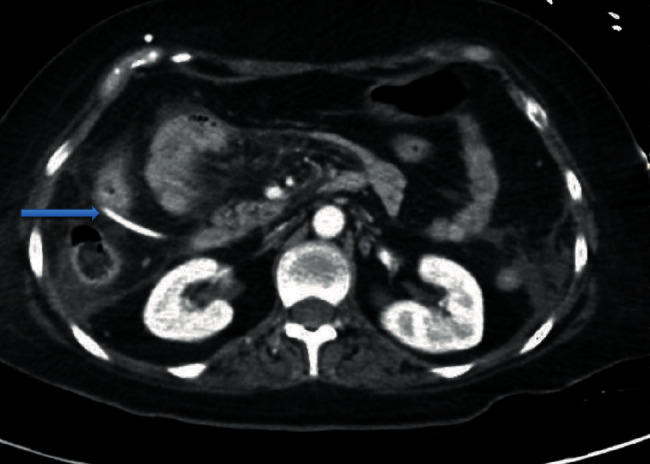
CT of the abdomen showing dilated loops of bowel concerning for enteritis and ventriculoperitoneal shunt (blue arrow).
